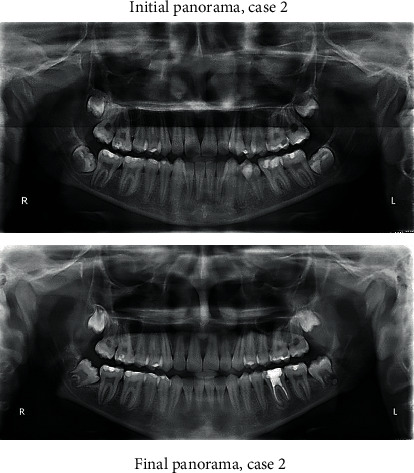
Initial and final panoramic radiographs.
